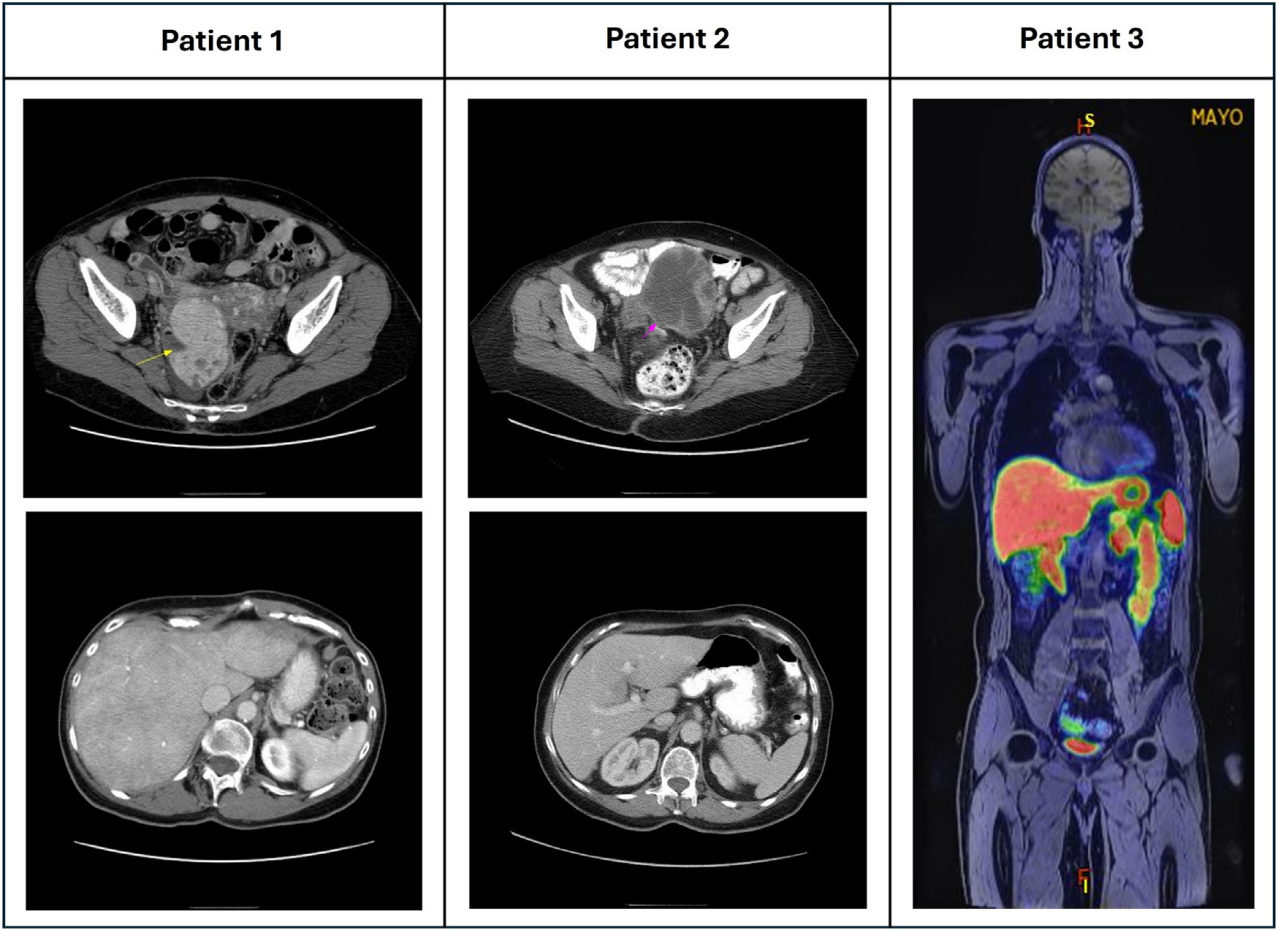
Computed tomography scans (*axial view*) of the ovarian tumors and nonaffected liver for the same individual at the time of cardiac surgery (patients 1 and 2). Gallium-68–labeled peptide positron-emission tomography scan showing a healthy liver in the patient with testicular tumor (resected at time of cardiac surgery).
